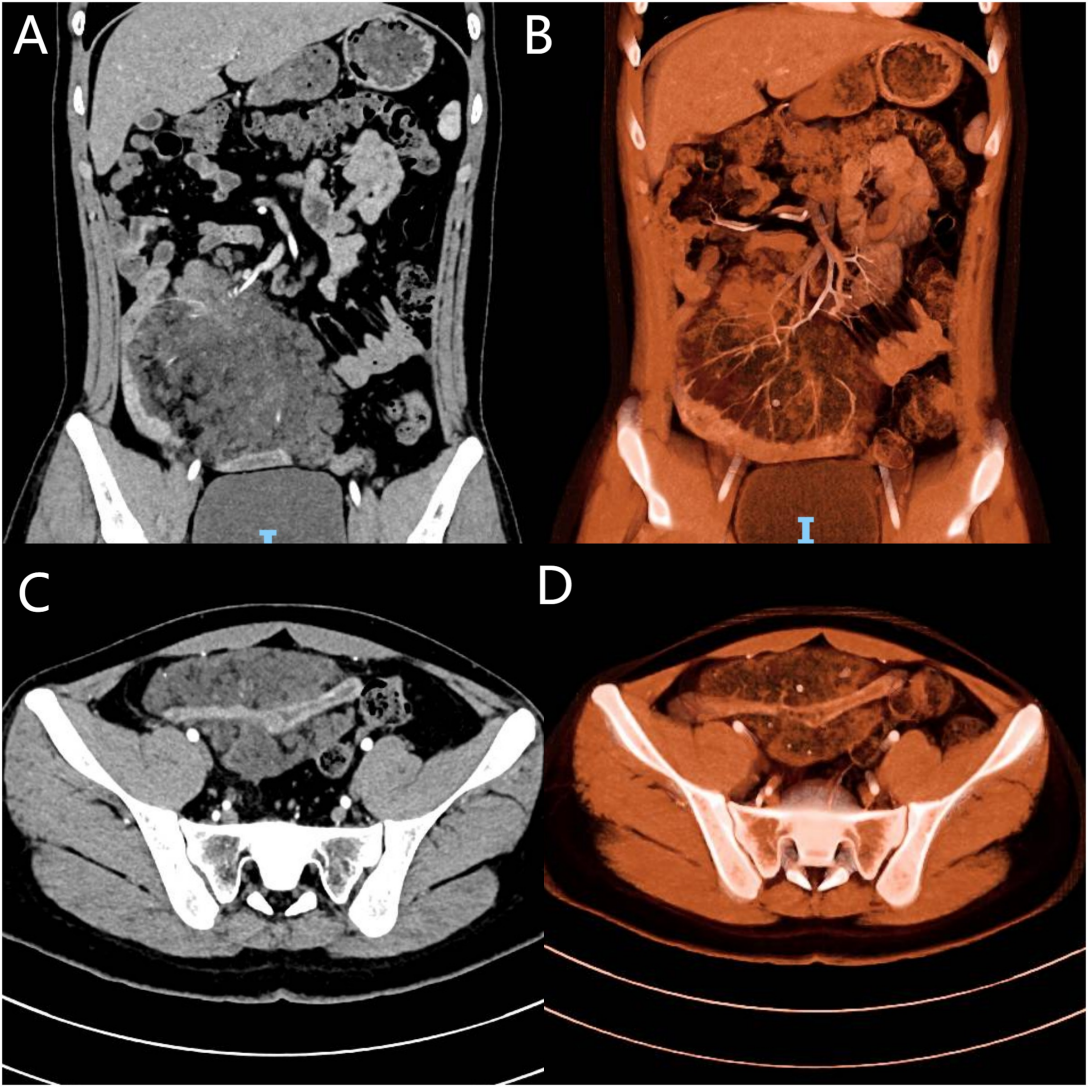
Contrast-enhanced CT and 3D reconstruction: (A) Coronal view showing a right lower abdominal mass originating from the mesentery. (B) Coronal 3D vascular reconstruction showing the tumor’s blood supply from the superior mesenteric artery. (C) Axial view showing the tumor encasing and invading the ileum. (D) Axial 3D reconstruction showing ileal involvement.
